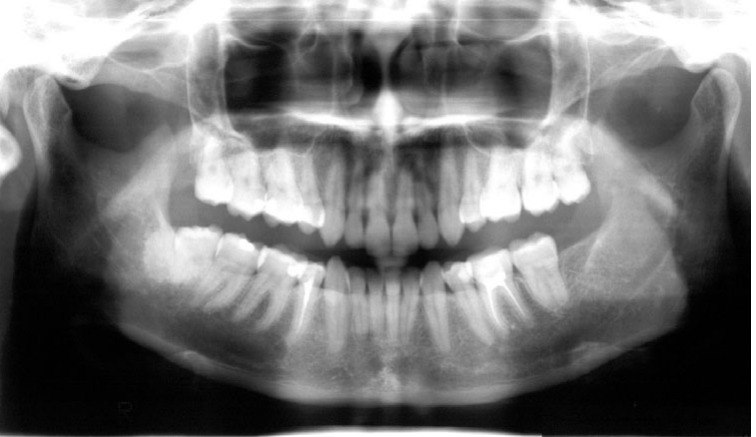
Follow-up panoramic X-ray taken one year after the operation indicates resolution of the lesion.
